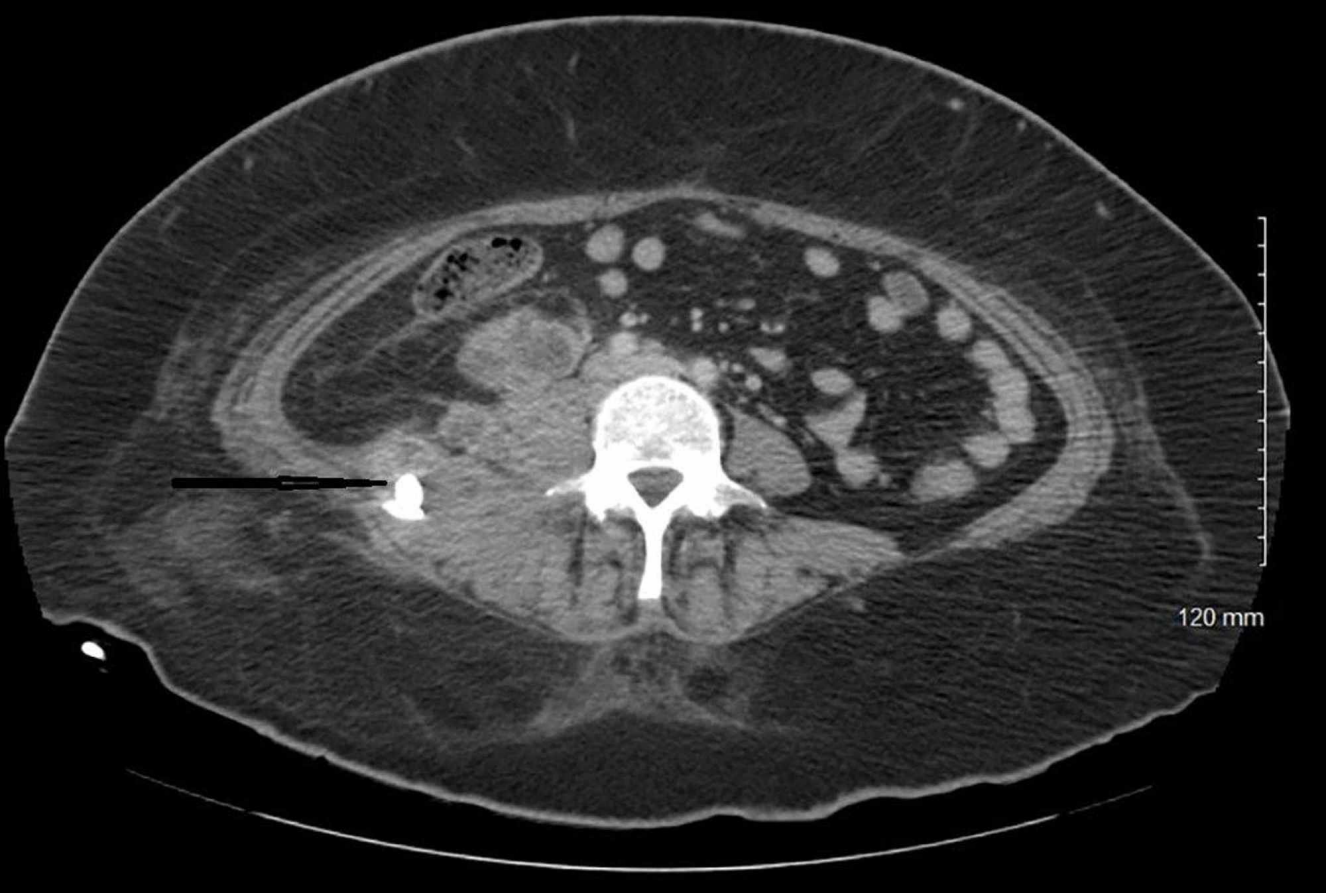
CT abdomen and pelvis with contrast after drainage and six days of antifungals Interval decrease in the size of the abscess at the site of the pigtail catheter placement CT: computed tomography
